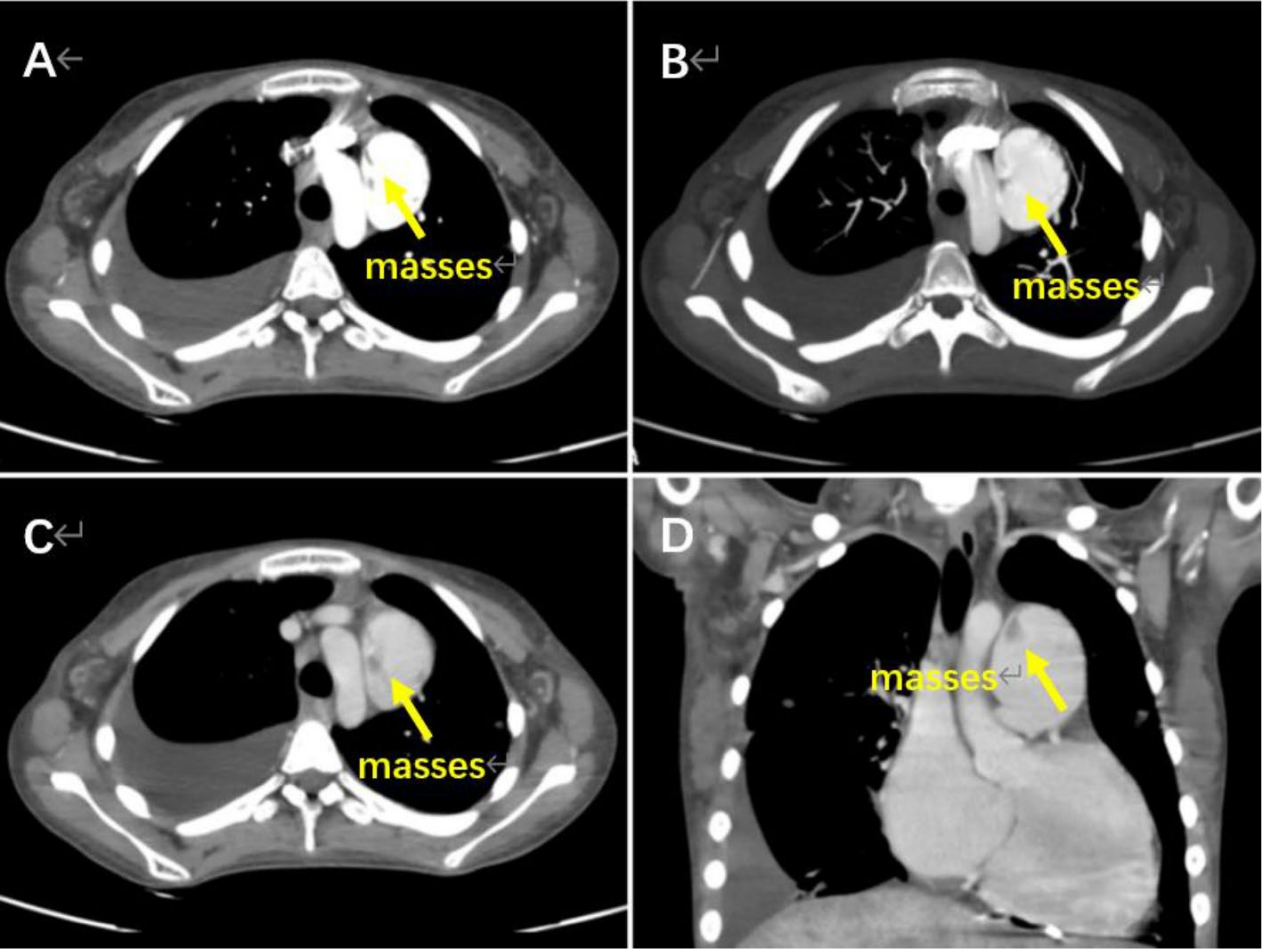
CTA images of case 2. Several masses in the dilated pulmonary artery (arrow)
